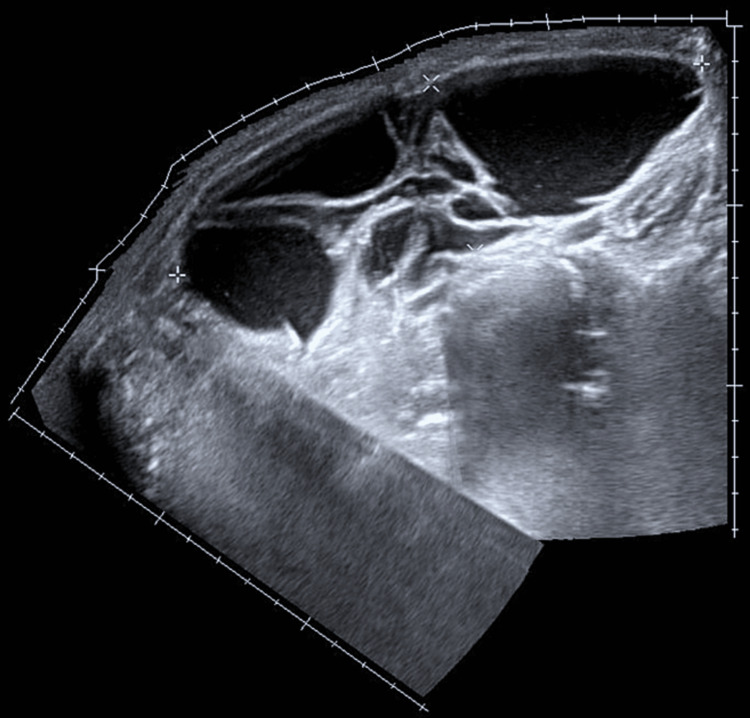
Sagittal abdominal ultrasound image demonstrating multiple large multiloculated, septated cystic structures.
